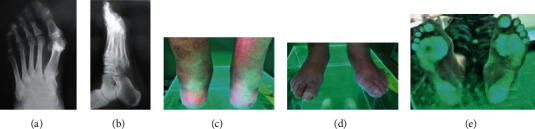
(a–f) Results of imaging tests (X-ray) and podoscopic tests: patient no. 6. (a) Dorsal-plantar foot X-ray: degeneration of the I head of metatarsal bones, phalangeal valgity, and overload lesions in metatarsophalangeal joints. (b) Lateral foot X-ray: enthesopathy of the plantar fascia and inflammation in the metatarsophalangeal joints. (c) Posterior photo: ankle joint and hindfoot evaluation. (d) Anterior picture of the feet: assessment of toe positioning and deformation. (e) Plantar photo on the podoscope: assessment of plantar part of the foot and height measurement of the longitudinal arch.
